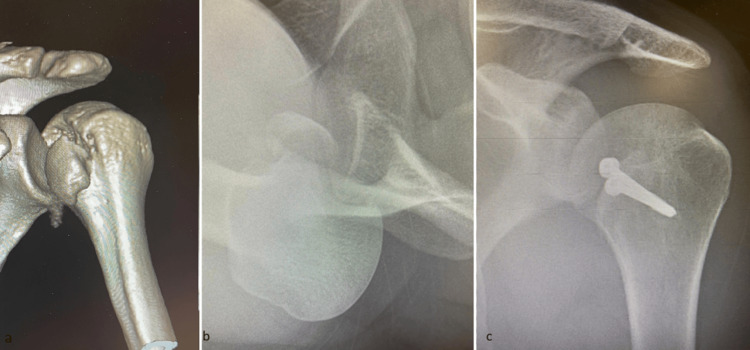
PSFD managed with the modified McLaughlin procedure (a) 3D CT reconstruction showing a posterior fracture-dislocation with reverse Hill-Sachs defect. (b) Preoperative axillary radiograph demonstrating a locked PSFD with associated humeral head impaction. (c) Postoperative radiograph showing restoration of joint congruency following the modified McLaughlin procedure. PSFD, posterior shoulder fracture-dislocation
